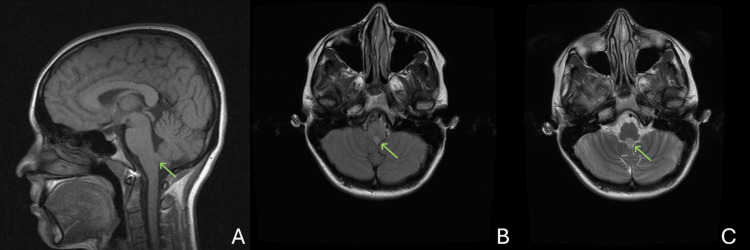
Initial MRI demonstrating a small fourth-ventricular lesion A. Sagittal T1-weighted image showing a 7 x 7 x 11 mm, well-circumscribed nodule along the floor of the fourth ventricle (arrow), without mass effect. B. Axial T1-weighted image demonstrating iso- to mildly hypointense signal of the lesion relative to gray matter (arrow). C. Axial T2-weighted postcontrast image showing mild hyperintensity and subtle but present mild enhancement of the lesion (arrow), without surrounding edema or hydrocephalus.
